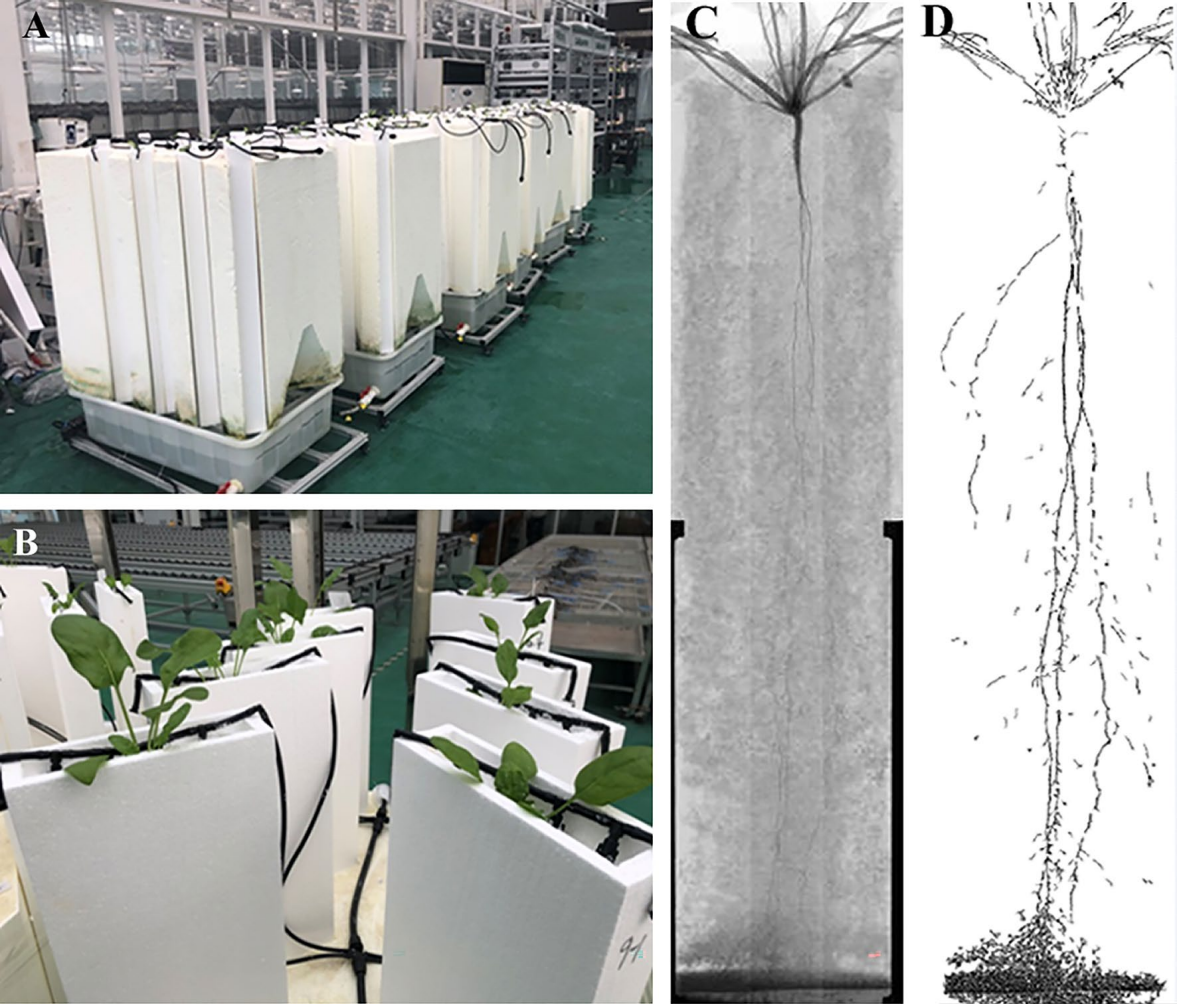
The rhizoboxes of the RootViz FS system in a greenhouse (A), a close shot of plants in the rhizoboxes (B), and an example of plant roots scanned by X-ray computed tomography without (C) and with filters (D).
